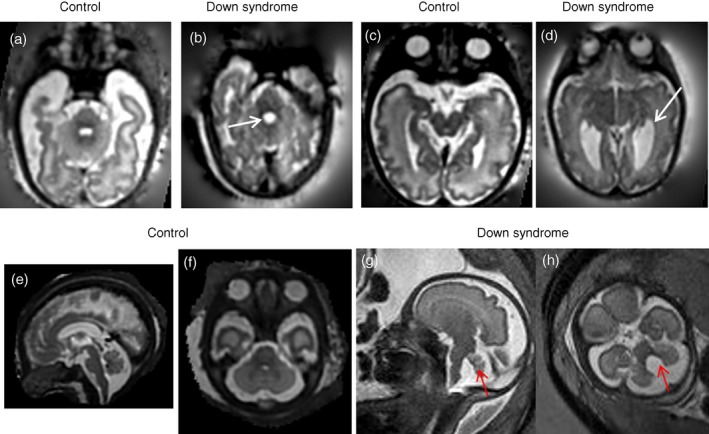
T2‐weighted fetal magnetic resonance imaging in a control fetus and a fetus with Down syndrome. T2‐weighted axial images showing the fourth (a,b) and lateral ventricle (c,d) in control (34^+1^
